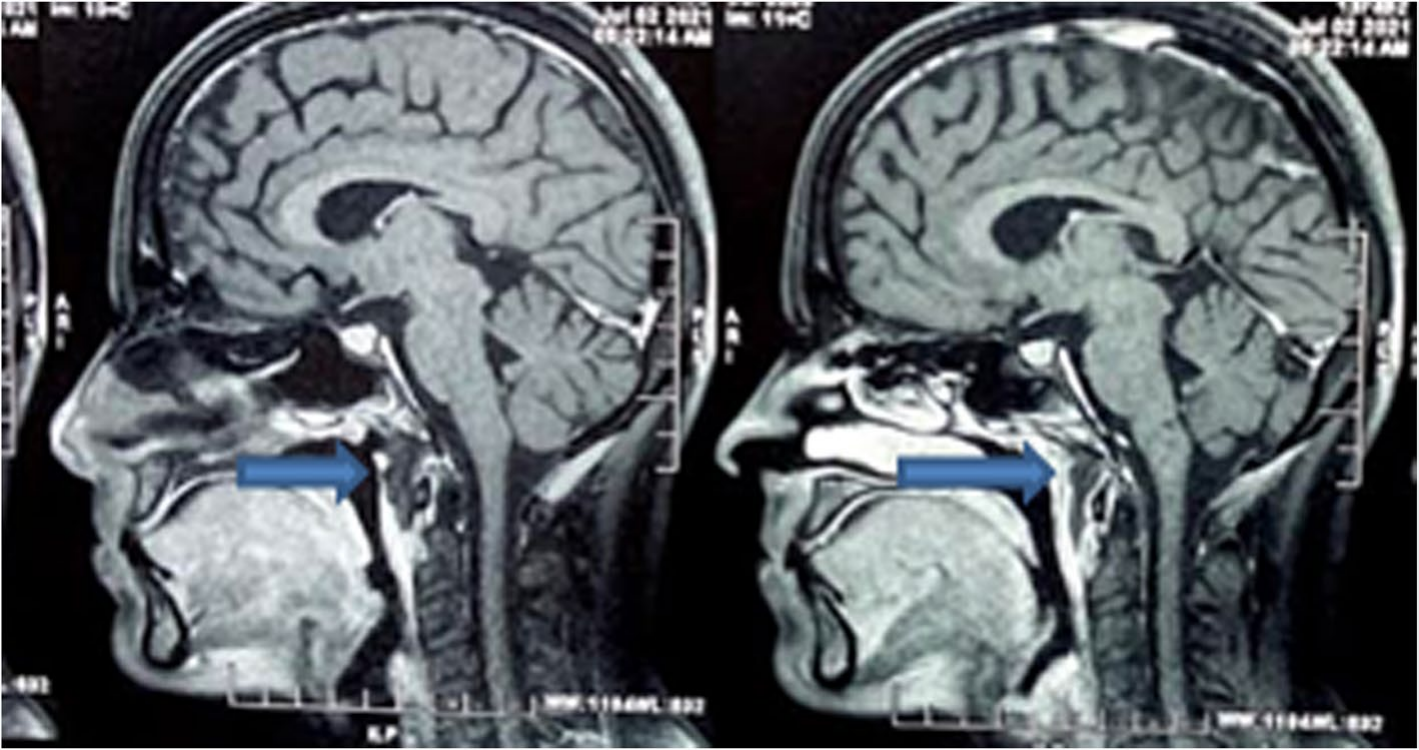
T1 contrast MRI image (Sagittal section) showing retropharyngeal abscess with no contrast uptake
